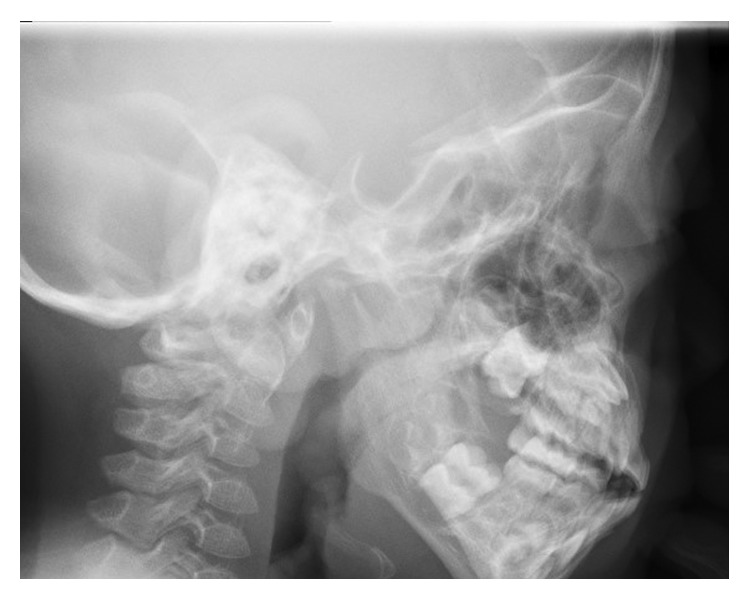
Lateral neck X-ray. Obstruction of the nasopharyngeal airway due to adenoid hypertrophy is apparent.
